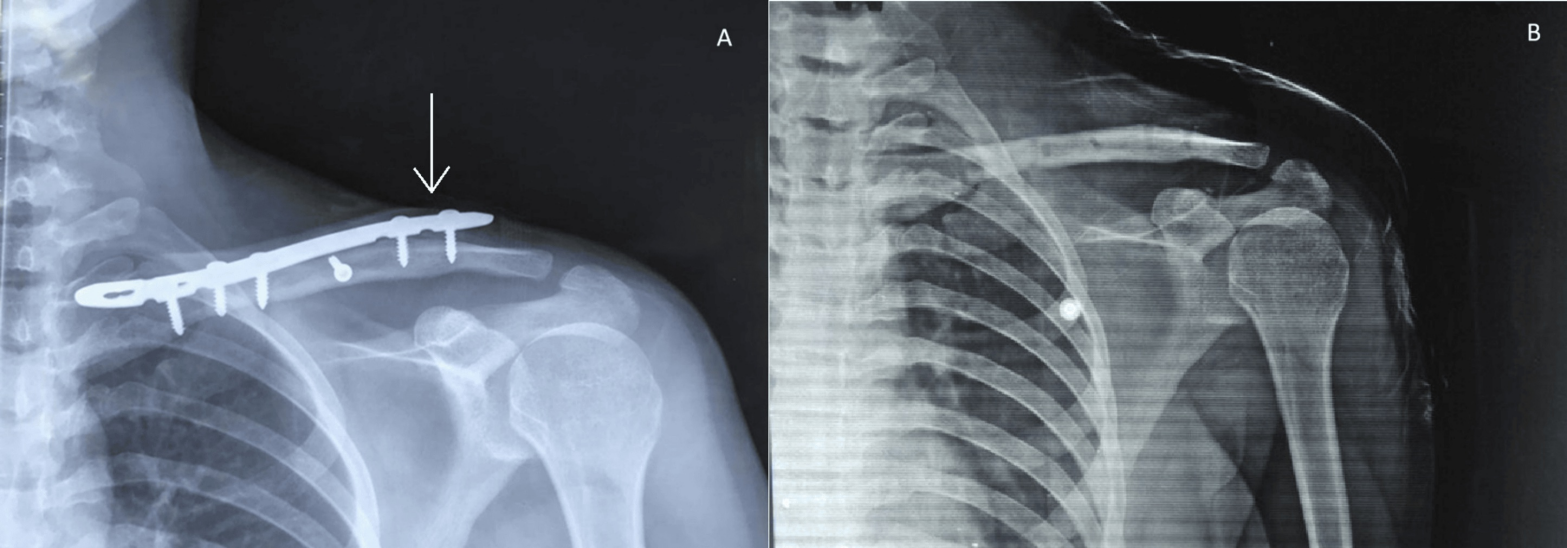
Plate pullout following fracture union necessitating removal of metalwork. (A) Nine months post-operative x-ray when the patient presented with complaints of hardware irritation (arrow depicting lateral pullout). (B) Post-operative x-ray following removal of metalwork.
